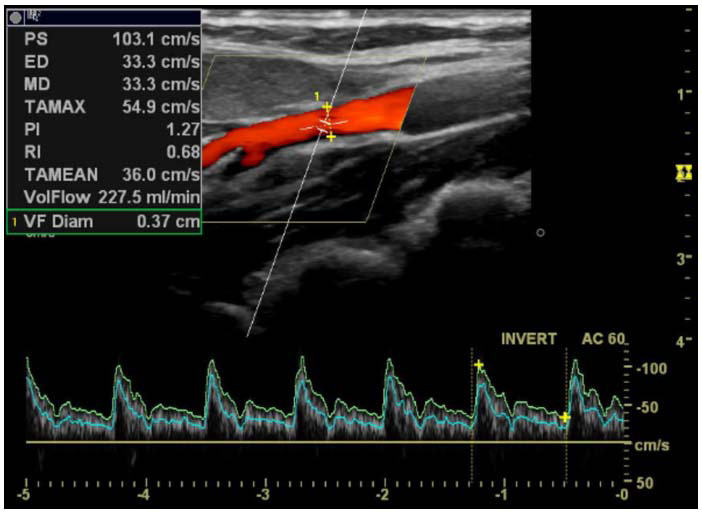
Left ECA duplex ultrasonography image, showing PS, ED, PI, RI, vessel diameter, and flow volume values in the headache-free period.
